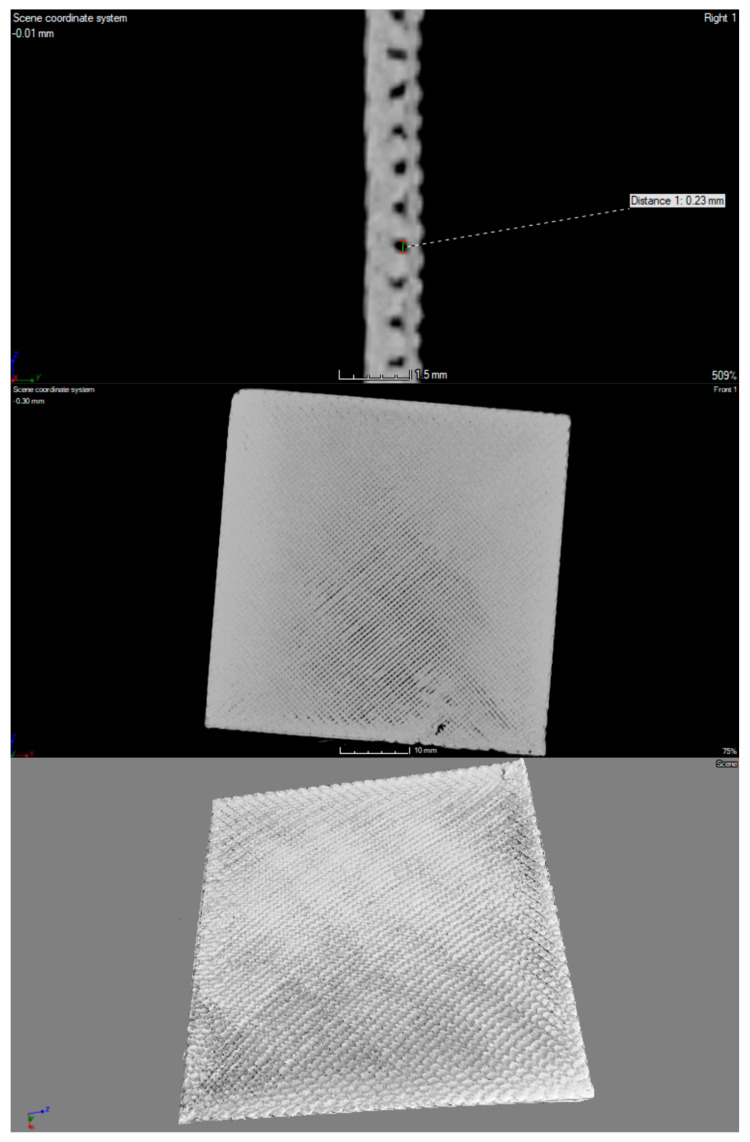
Micro CT imaging of 3D printed porous standard plate modified with rhodamine B derivative.
